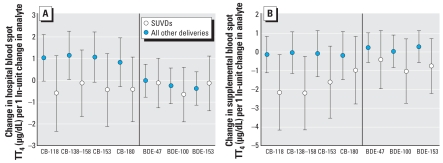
Change in thyroid hormones measured in blood spots associated with a 1 ln-unit change in analyte by birth delivery mode. (*A*) Hospital blood spot TT_4_ (SUVD = 86; all other deliveries = 179). (*B*) Subsequent blood spot TT_4_ (SUVD = 35; all other deliveries = 104). All models adjusted for baby’s sex, gestational age, maternal age, maternal race, maternal prepregnancy BMI, smoking status, and time since birth that blood spot was collected (in days). Error bars represent 95% CIs.
